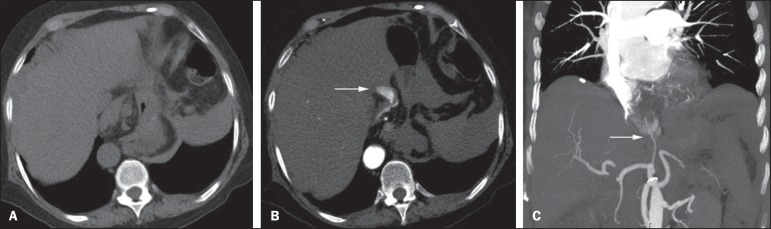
Non-contrast-enhanced CT angiography (A), in the arterial
